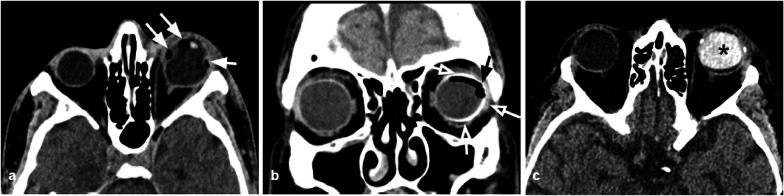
CT appearance of the various ocular interventions that can mimic open globe fractures and/or vitreous hemorrhages. 32-Year-old patients with an increase of the anteroposterior diameter of the left eyeball, related to a myopic eye, previously treated for e retinal detachment, with current CT evidence of intraocular air-bubble-like components (white arrows), corresponding to perfluoropropane gas bubbles (a). b Coronal CT reconstruction showing a scleral buckle surgery with low attenuation device (black arrow) that mildly indents the temporal aspect of the left globe and encircles the scleral buckle (metallic density ring around the left globe, white empty arrows). c. highly attenuation silicone oil in the left globe (asterisk) placed after surgical vitrectomy to treat a retinal detachment. To help the differential diagnosis with hemovitreous, the attenuation values in Hounsfield Units (HU) can be assessed, with silicone oil having values greater than 100 HU
